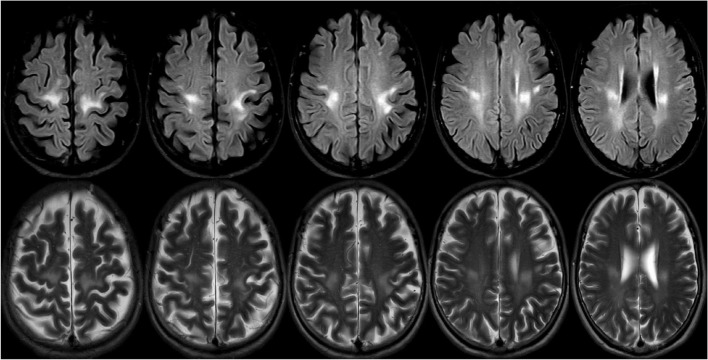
A 69-years-old female presented with a 2-year history of progressive weakness of the lower limbs. A brain magnetic resonance imaging (MRI) revealed abnormal T2 and flair hyperintensities in the periventricular and deep cerebral white matter, and the bilateral subcortical areas of parietal white matter (the lesions were continuous and symmetrically distributed). Reduced galactocerebrosidase activity and GALC gene confirmed the diagnosis of adult late-onset GLD.
